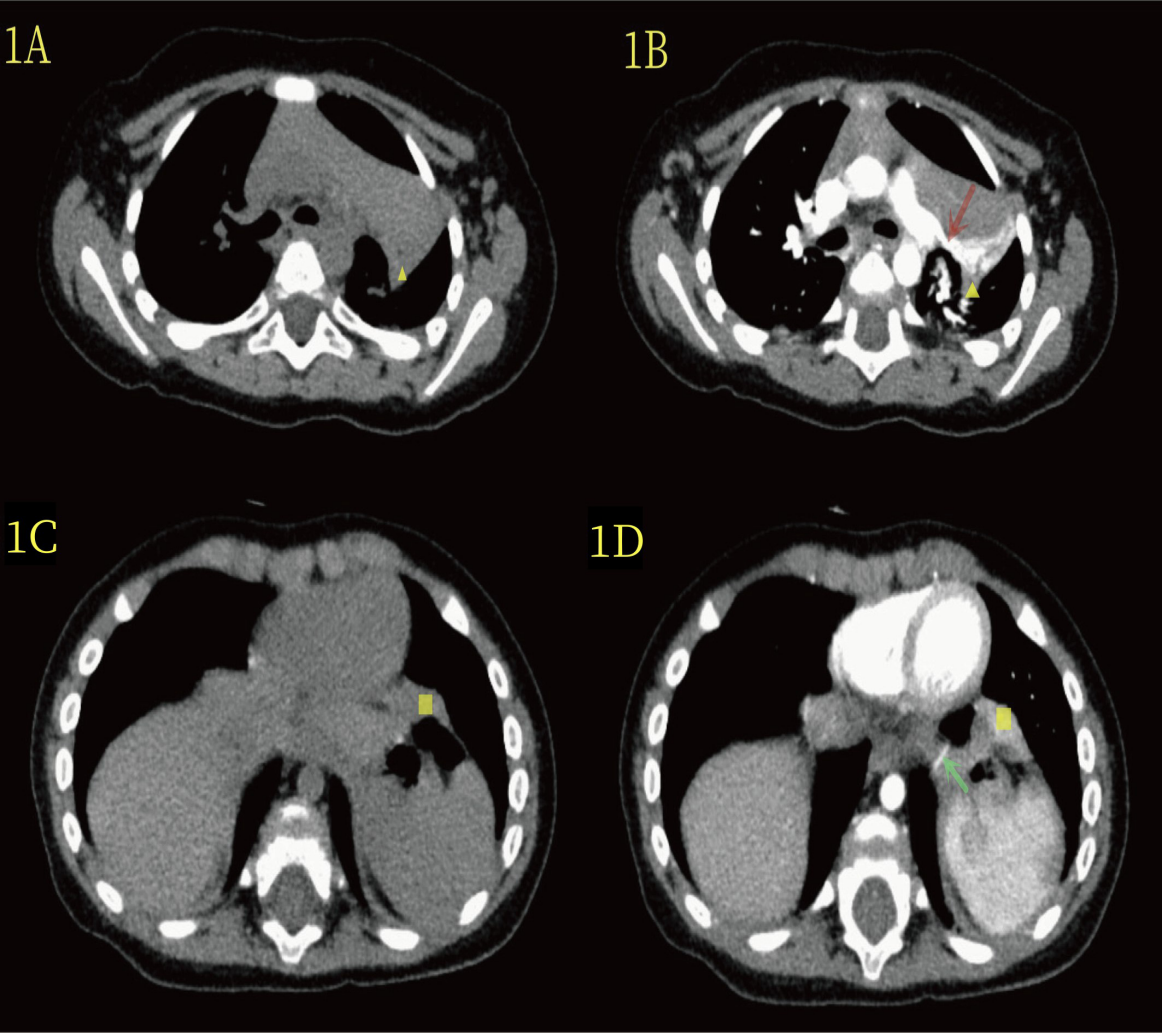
(A) The yellow triangle in the CT plain scan indicates the lesion at the upper hilum of the lung. (B) On enhanced CT scan, the yellow triangle highlights the lesion at the upper hilum of the lung, and the red arrow denotes the blood-supplying artery (branch of the upper pulmonary artery). (C) In the CT plain scan, the yellow quadrilateral indicates the lesion in the lower diaphragm. (D) On enhanced CT, the yellow quadrilateral marks the lesion in the lower diaphragm, and the green-clip head highlights the blood-supplying artery (abdominal aorta).
